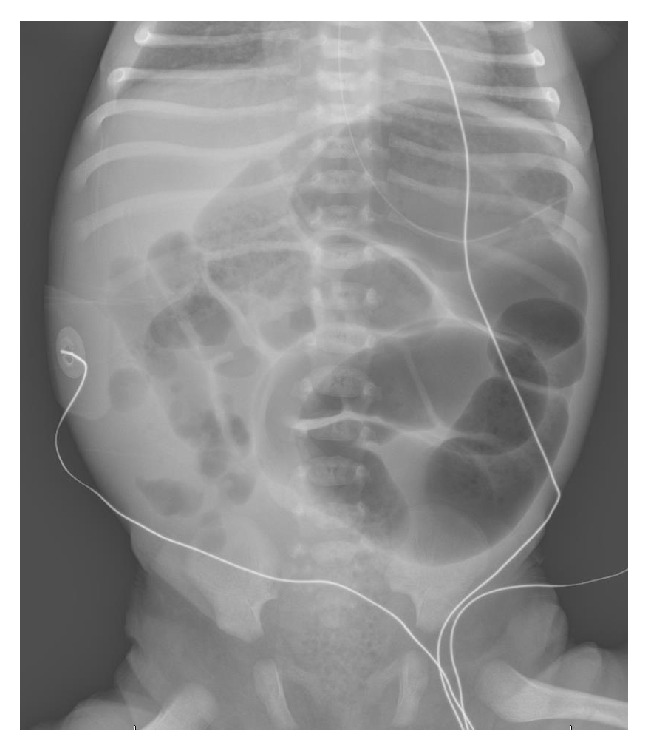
Abdominal radiograph obtained on HD 2 demonstrated dilation of the proximal bowel with normal caliber distal bowel and a paucity of air in the rectum.
